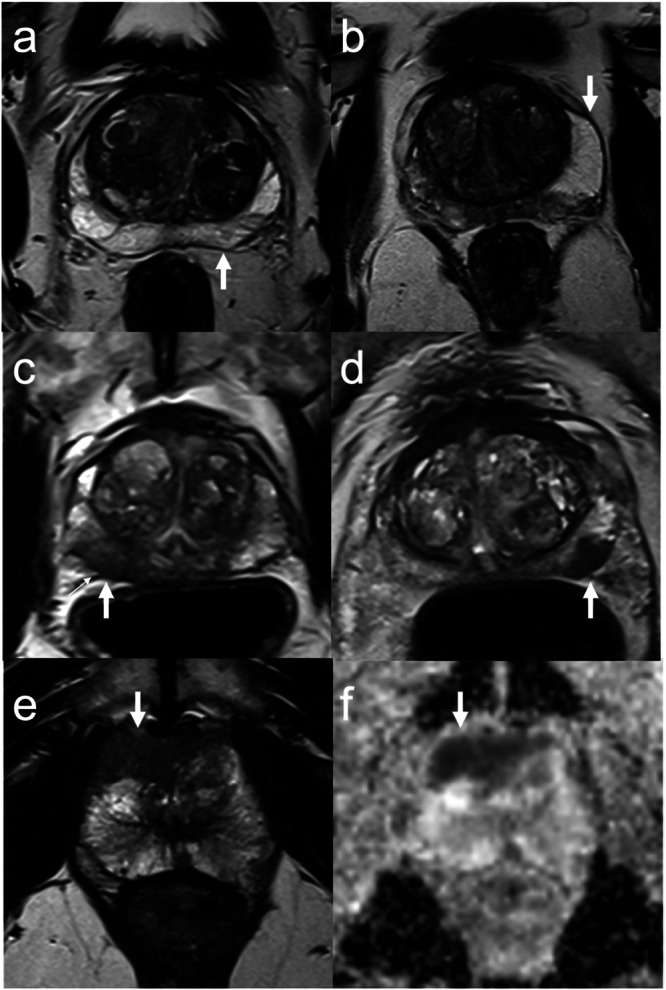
MRI-derived parameters for extraprostatic extension prediction in MRI T-staging. A low-signal-intensity band on T2-weighted images represents the so-called prostate “pseudocapsule”. This pseudocapsule is more distinct at the dorsal side of the peripheral zone (arrow) (a) than at the ventral side of the transition zone (arrow) (b). Irregularity (arrow) (c) and bulging (arrow) (d) of the pseudocapsule due to likely cancerous tissue in the dorsal peripheral zone can be assessed on high-quality T2-weighted images. In MRI T-staging, this focal breach of either mrT3a or mrT2 disease is challenging to correctly predict focal pT3a or pT2a. Irregularity of the pseudocapsule may be assessed as focal extraprostatic extension (focal mrT3a), while bulging of the pseudocapsule may be assessed as organ-confined disease (mrT2), despite the higher risk of focal extraprostatic extension at pathology (focal pT3a). Clear disruption of the pseudocapsule at the anterior border of the transition zone, combined with frank extracapsular breach (arrow) on T2w-images (e) and diffusion weighted images (f), should be assessed as established extraprostatic extension (established mrT3a). Pathology assessment showed established pT3a
